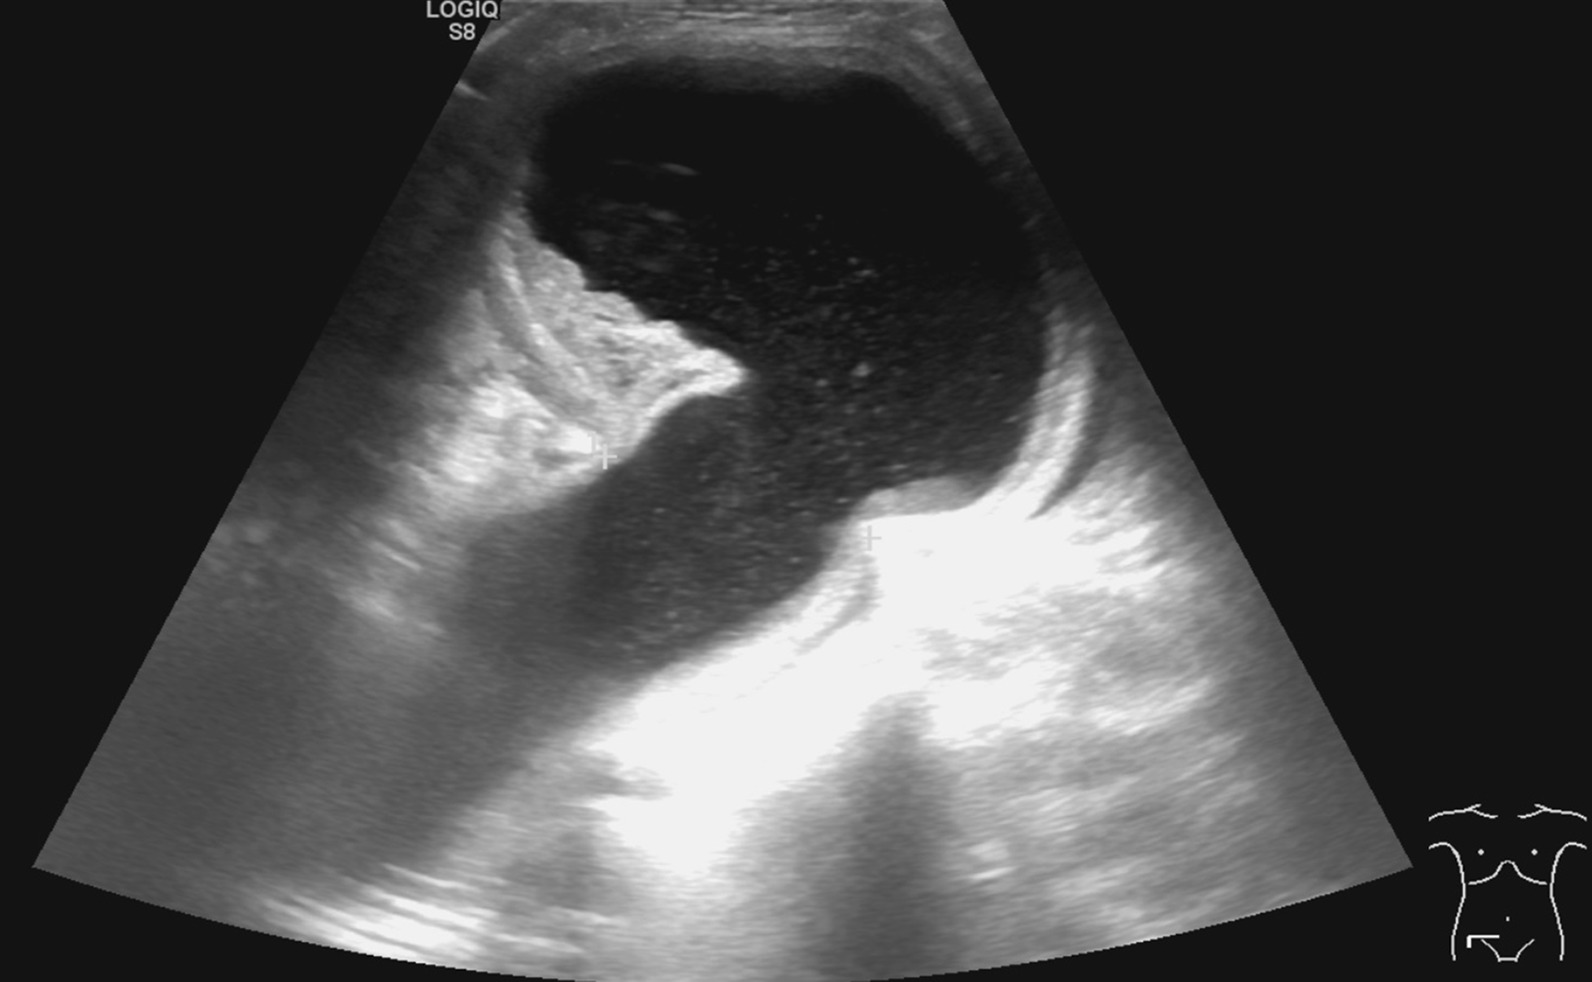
Preoperative abdominal ultrasound showed an oedematous intestinal loop in a 70-mm-long hernial sac protruding via a 30-mm hernial orifice
